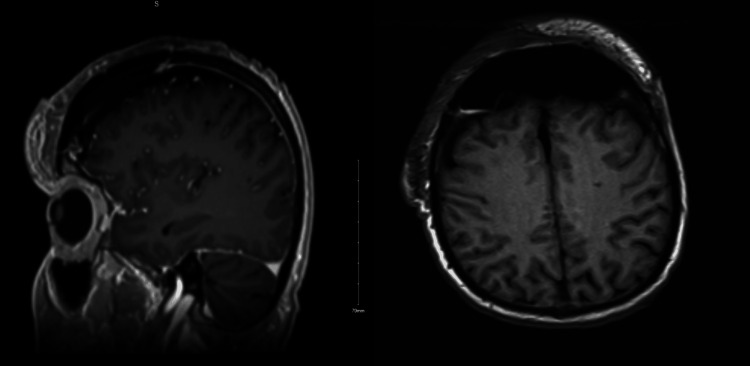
Sagittal and axial MRI views of the brain showing resection of the fronto-parietal mass, placement of a craniplasty graft, and postoperative pneumocephalus
